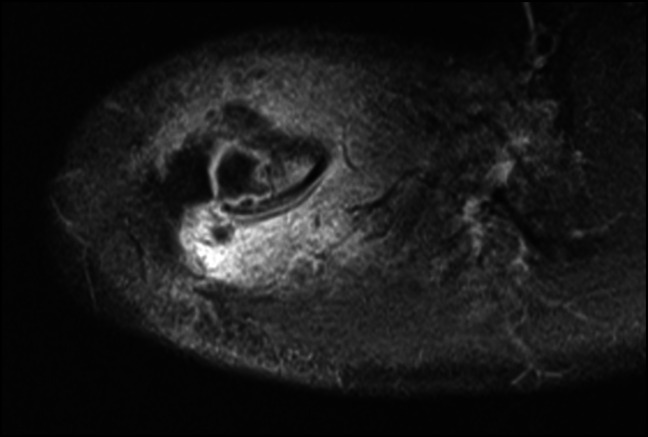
Axial postcontrast T1-weighted MRI demonstrating penumbra sign with cloaca.
